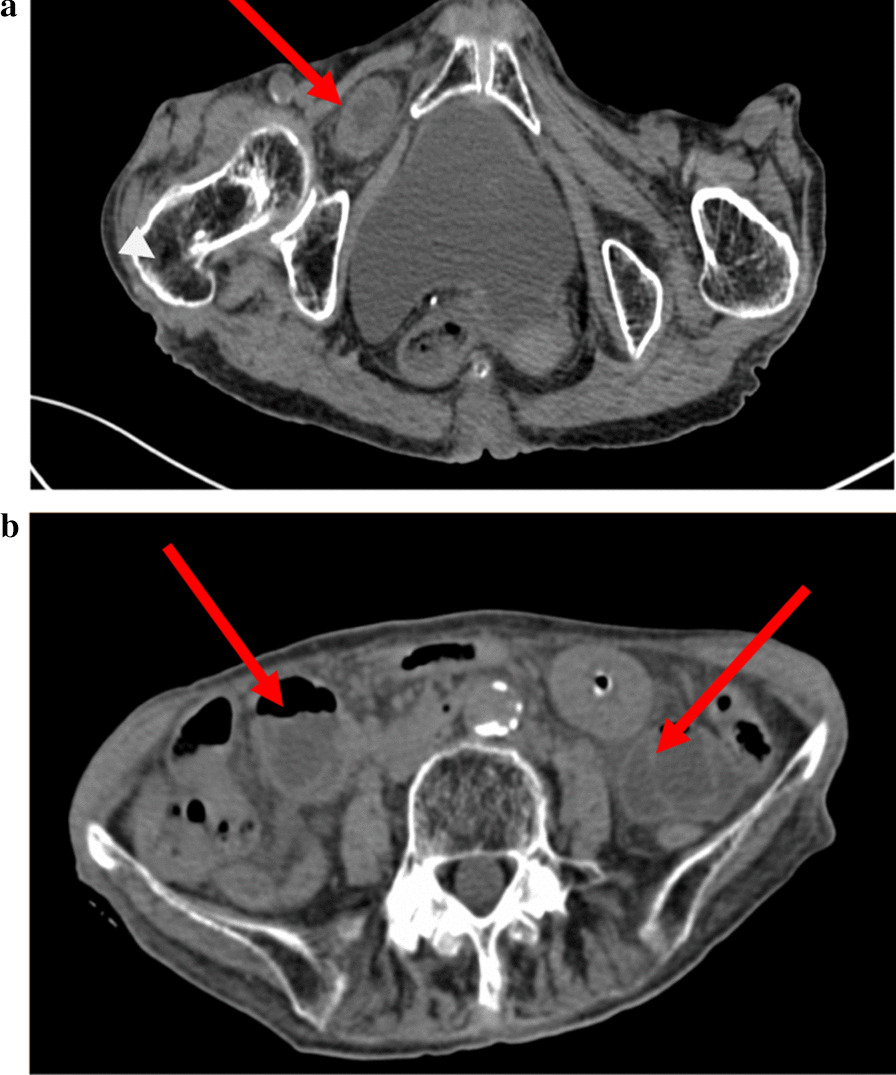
a Abdominal CT showing small bowel herniated into the right obturator canal. b Abdominal CT showing the dilated small bowel loops above the site of obstruction
